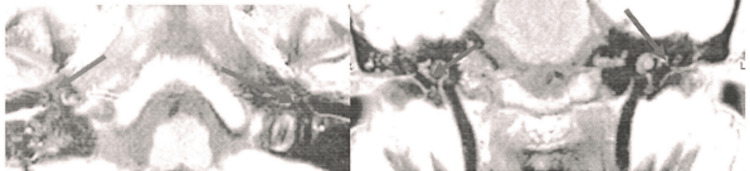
Representative MRI images of a treated patient indicating VELNEZ degradation The patient underwent right ear type I tympanoplasty. The right external auditory canal shows normal aeration. There is normal expected signal void in the right middle ear cavity and right mastoid antrum. The left tympanic membrane (neo-tympanum) is normal in appearance and there is no evidence of any residual soft tissue or fluid density in the middle ear.
